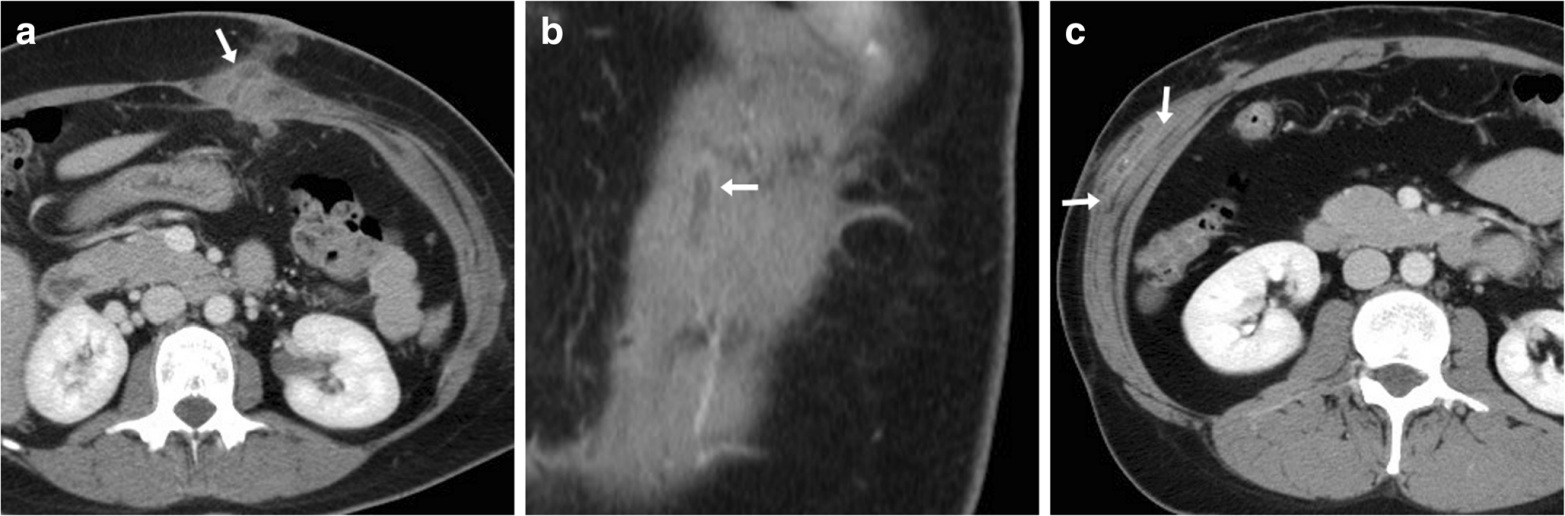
a, b Usual CT appearance of a trocar access site following uncomplicated laparoscopic cholecystectomy. Axial (a) and coronal (b) images show a tubular non-enhancing hypodense structure (*arrows*) crossing through the mildly thickened left rectus abdominis muscle. This normal finding should be differentiated from c, which is an intraparietal abscess (*arrows* in c) developing within the right external and internal oblique muscles following laparoscopic trocar access and manifesting with local swelling
